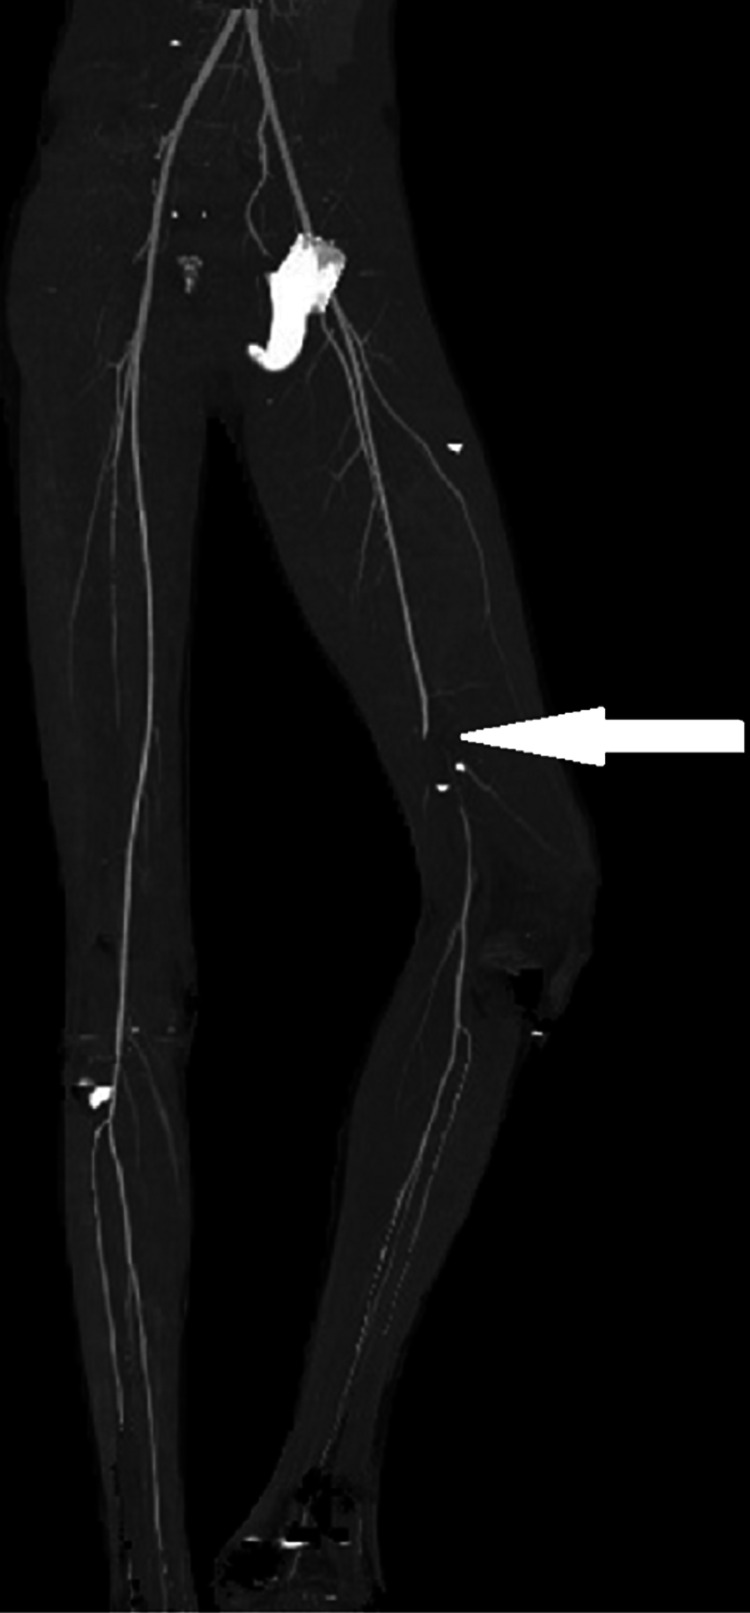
Computed tomography angiography (CTA) of the lower limb performed preoperatively showing an intimal rupture of the right superficial femoral artery with interruption of the blood flow (white arrow)
